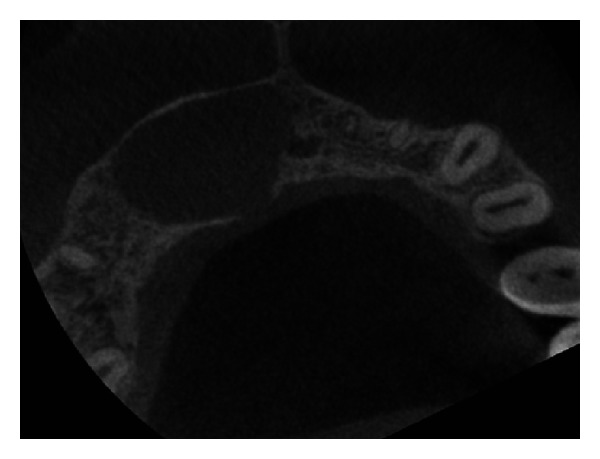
Pre-operative CBCT image in axial plane.
